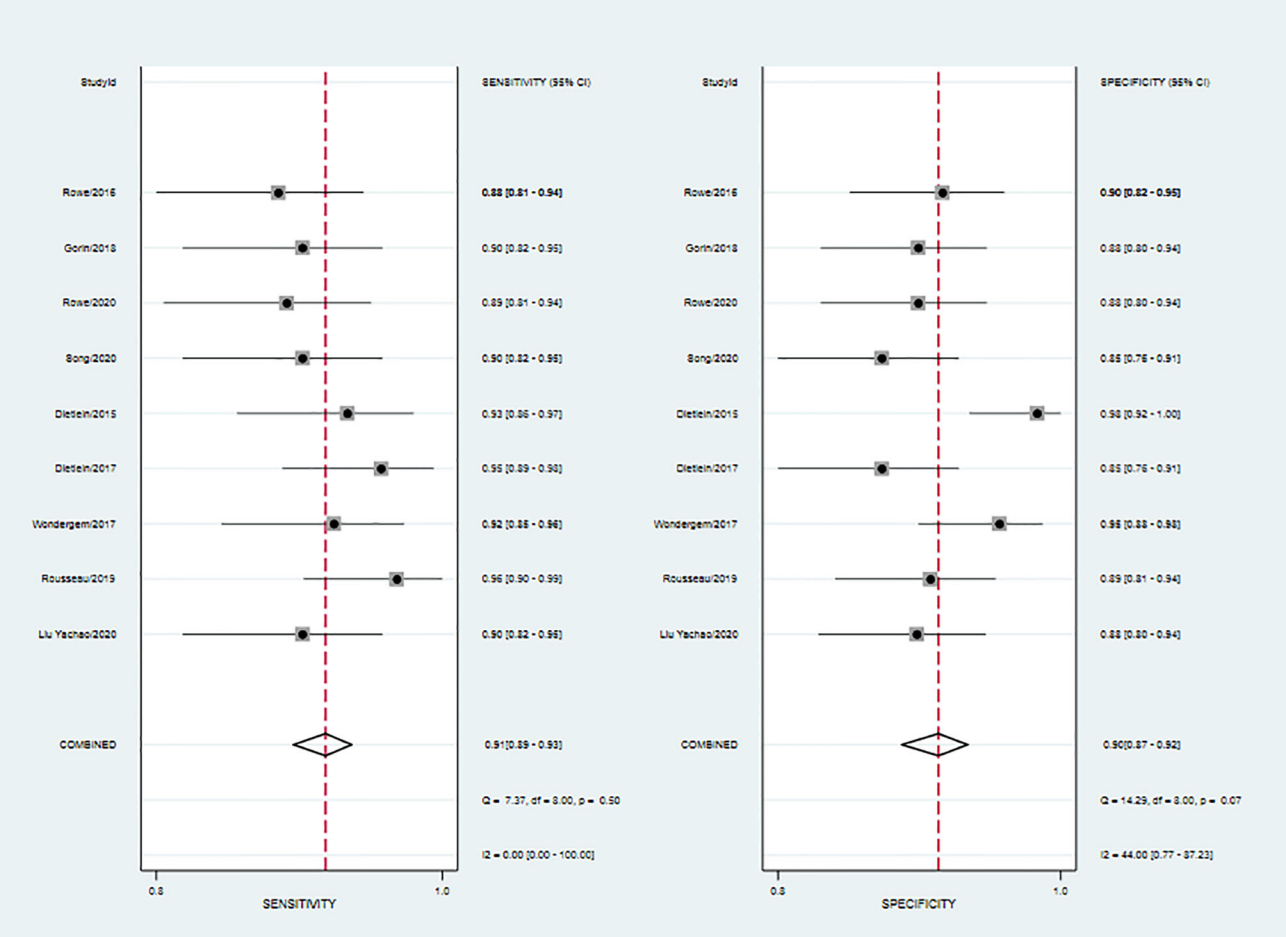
Forest map of 18F-DCFPyL PSMA PET/CT.
